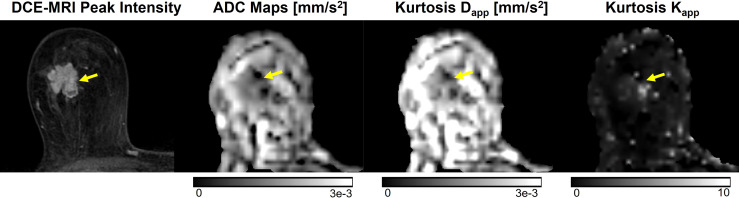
Example of DKI analysis using b-values of 0, 500, 1000, and 2000 s/mm^2^, compared to conventional ADC images using b-values of 0 and 1000 s/mm^2^ and DCE-MRI peak intensity subtraction (1 min 30 s post-contrast). Invasive ductal carcinoma in a 67-year-old patient is indicated by the yellow arrow. The lesion displays higher mean kurtosis (K_app_) than surrounding healthy tissue. Images were acquired using a wide-bore 3T scanner, and mean kurtosis and diffusivity (D_app_) were calculated as previously demonstrated (181).
